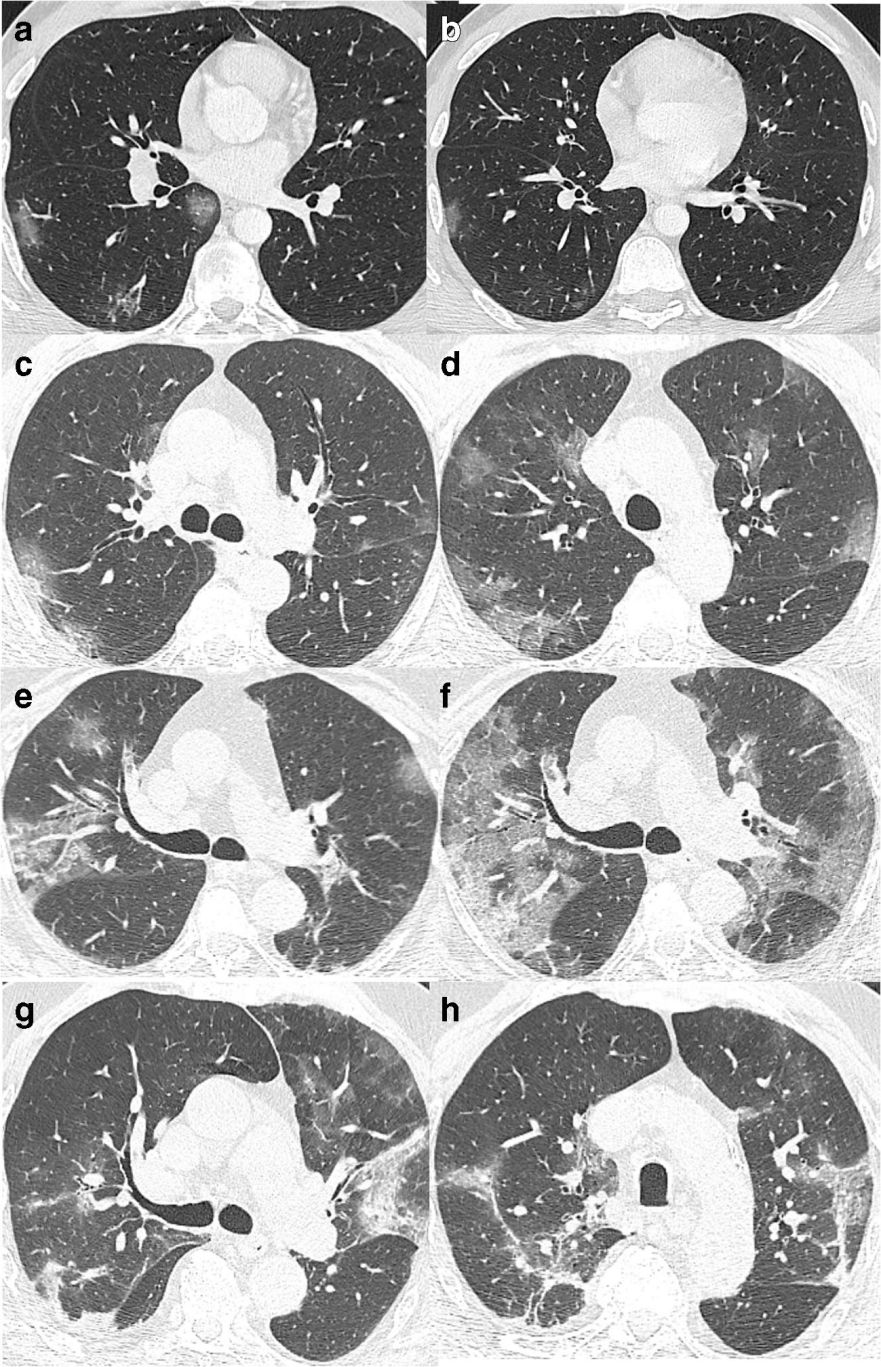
Appearance of COVID-19 on CT*.*
